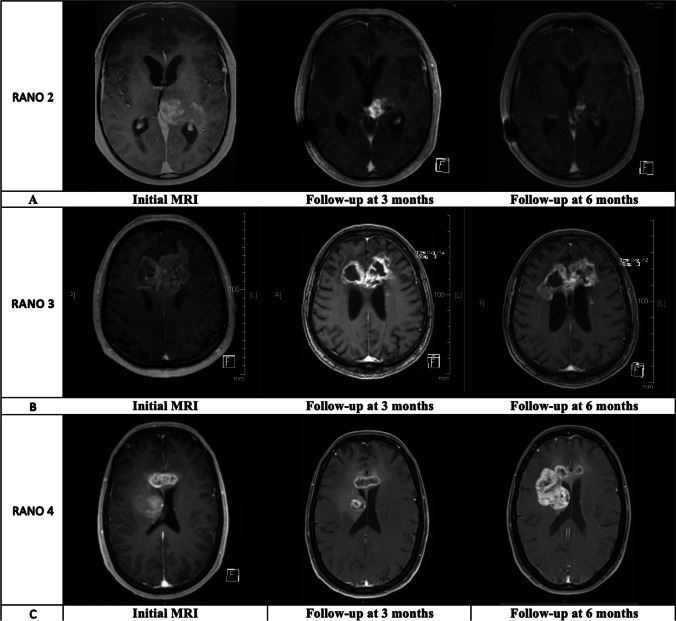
Examples of good and poor response to tumor treatment as seen on contrast-enhanced MRI and based on the RANO (response assessment in neuro-oncology) criteria, showing varying responses to treatment according to the RANO criteria comparing initial MRI with contrast with radiological response at follow-up at 3 and 6 months after tumor treatment: A partial radiological response (RANO 2), B stable disease (RANO 3), and C disease progression as defined by RANO 4
